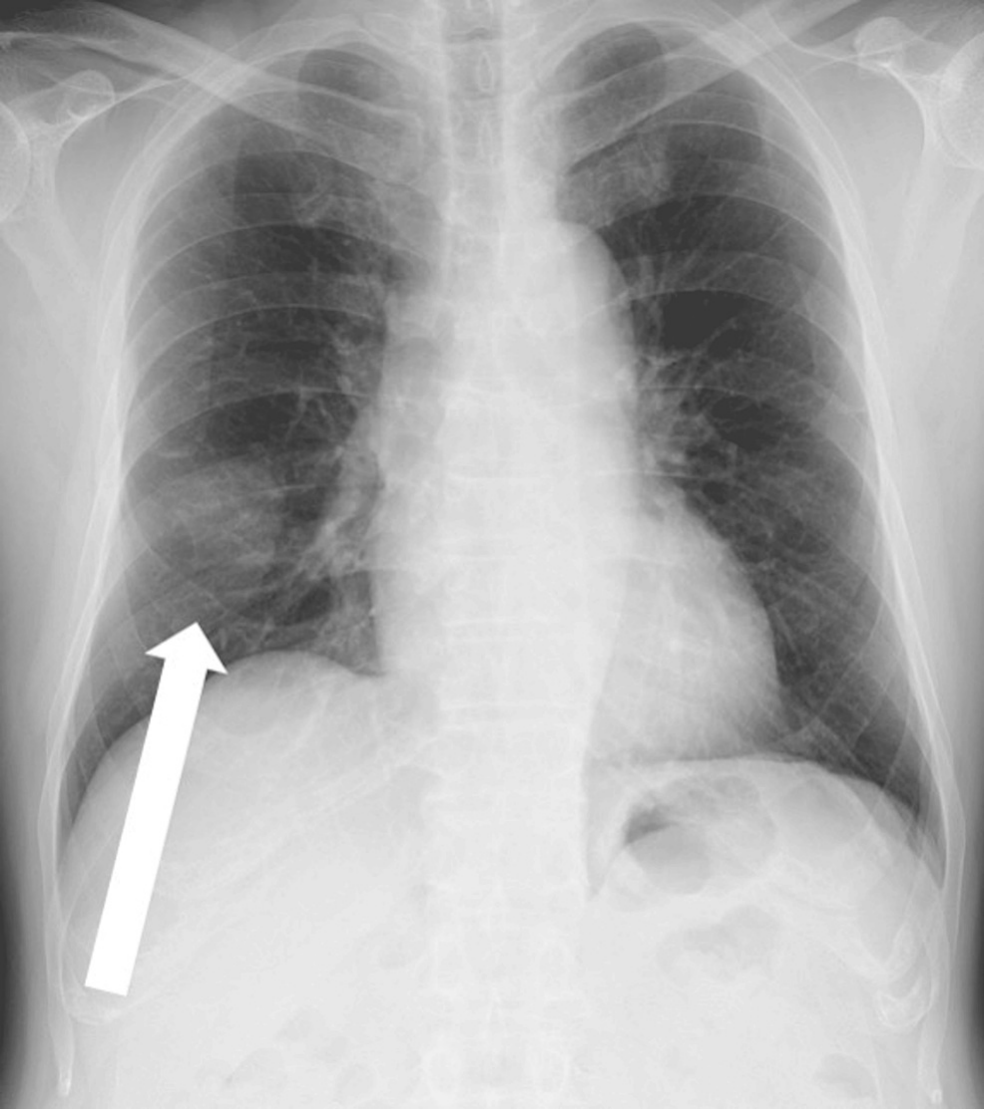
Chest radiograph (frontal view) A tumor shadow with indistinct boundaries in the right middle lung field.
